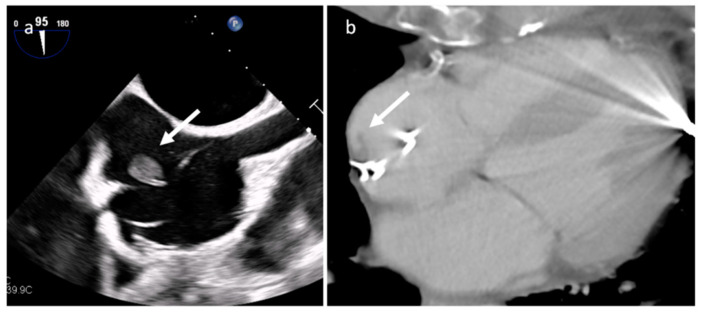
Electro-catheter endocarditis at transesophageal echocardiography (a) and CT (b).
